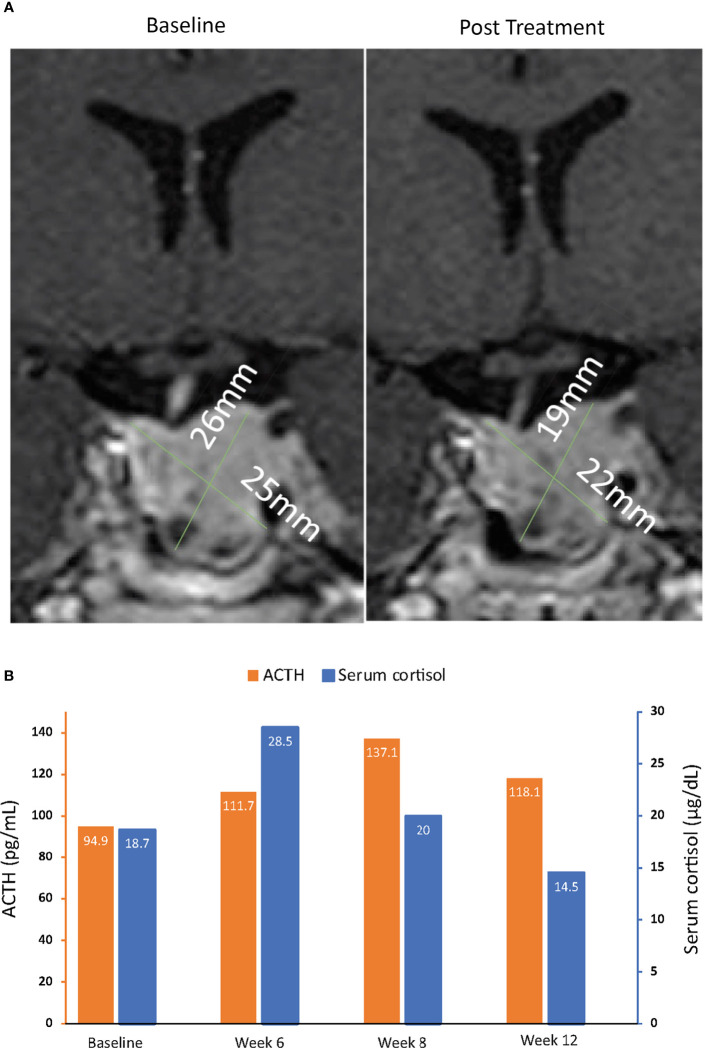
MRI of pituitary macroadenomas (A) and ACTH and cortisol levels (B) in case 4: a 43-year-old man with Cushing disease treated with relacorilant for 12 weeks. (A) Coronal post contrast T1-weighted MRI obtained at diagnosis (left image) after administration of gadolinium showed a pituitary macroadenoma measuring 22 × 25 × 26 mm with suprasellar extension, right displacement of the pituitary stalk, and invasion of the left cavernous sinus. The tumor was isointense to the gray matter and slightly inhomogeneous for the presence of cystic changes in its lower aspect. MRI of the hypophysis obtained within 12 weeks after the last dose of relacorilant (right image), after treatment with relacorilant, showed a reduction in the size of the macroadenoma (21 × 22 × 19 mm). (B) ACTH and cortisol levels. Normal laboratory ranges: ACTH, 6.0-50 pg/mL; serum cortisol, 4.6-20.6 µg/dL. To convert ACTH values from pg/mL to pmol/L, multiply by 0.22. To convert serum cortisol from µg/dL to nmol/L, multiply by 27.6. ACTH, adrenocorticotropic hormone.
